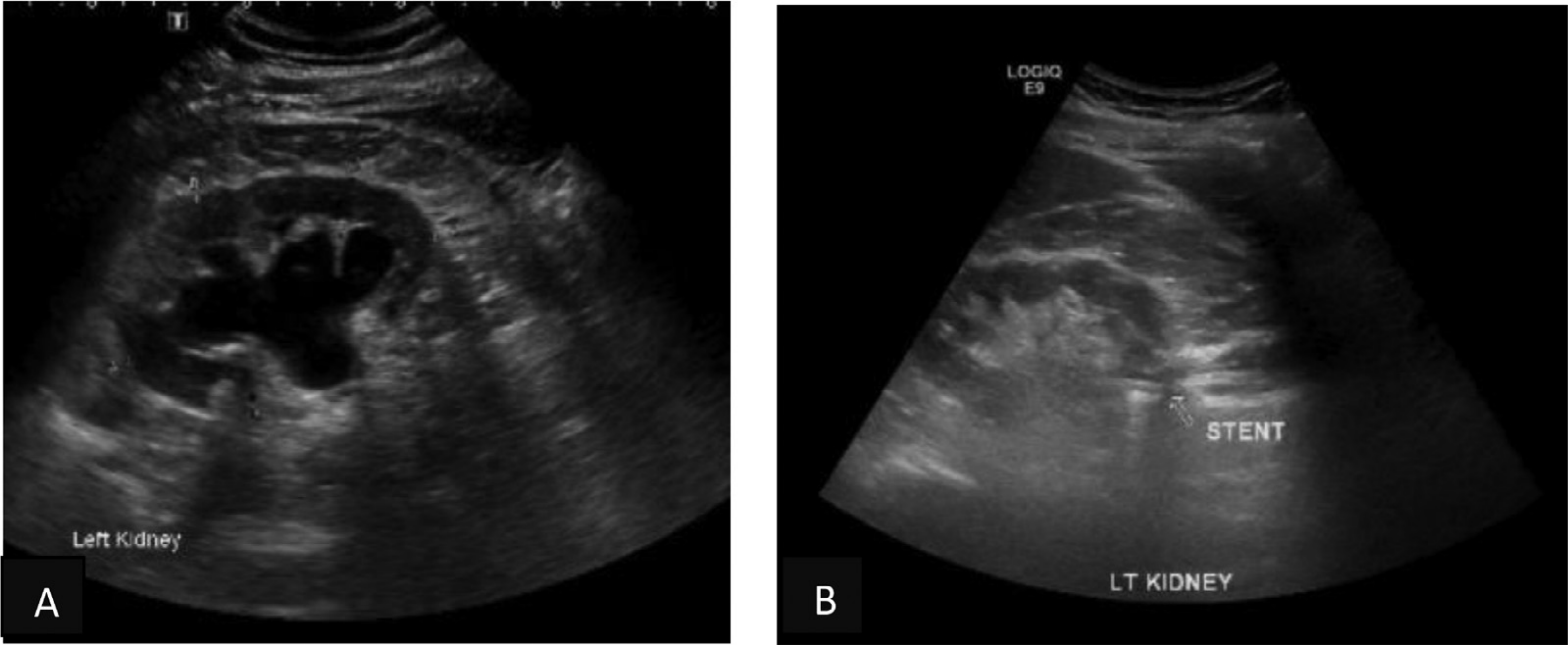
Ultrasound of left kidney, (A) hydronephrosis, (B) after Memokath insertion resolution of hydronephrosis.
